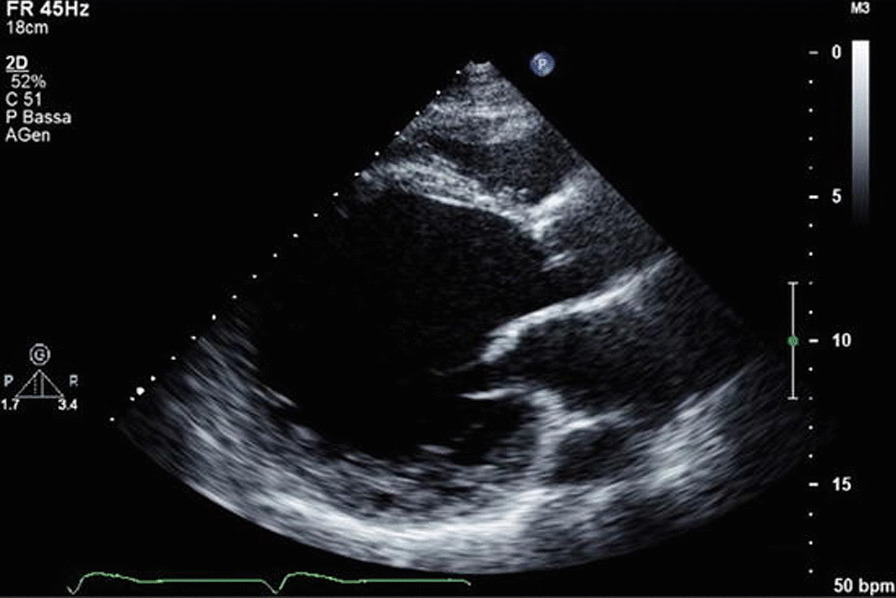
Two-dimensional echocardiogram showing evidence of dilated cardiomyopathy
